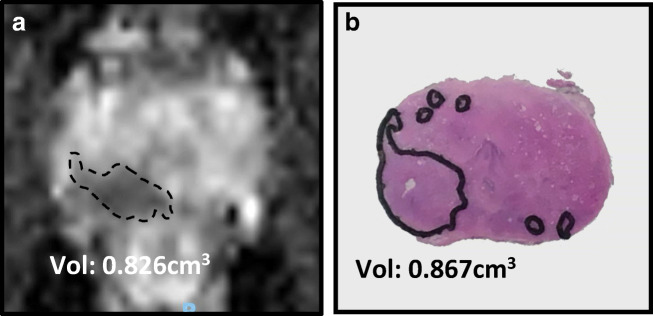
mp-MRI lesion volume compared with pathology volume
